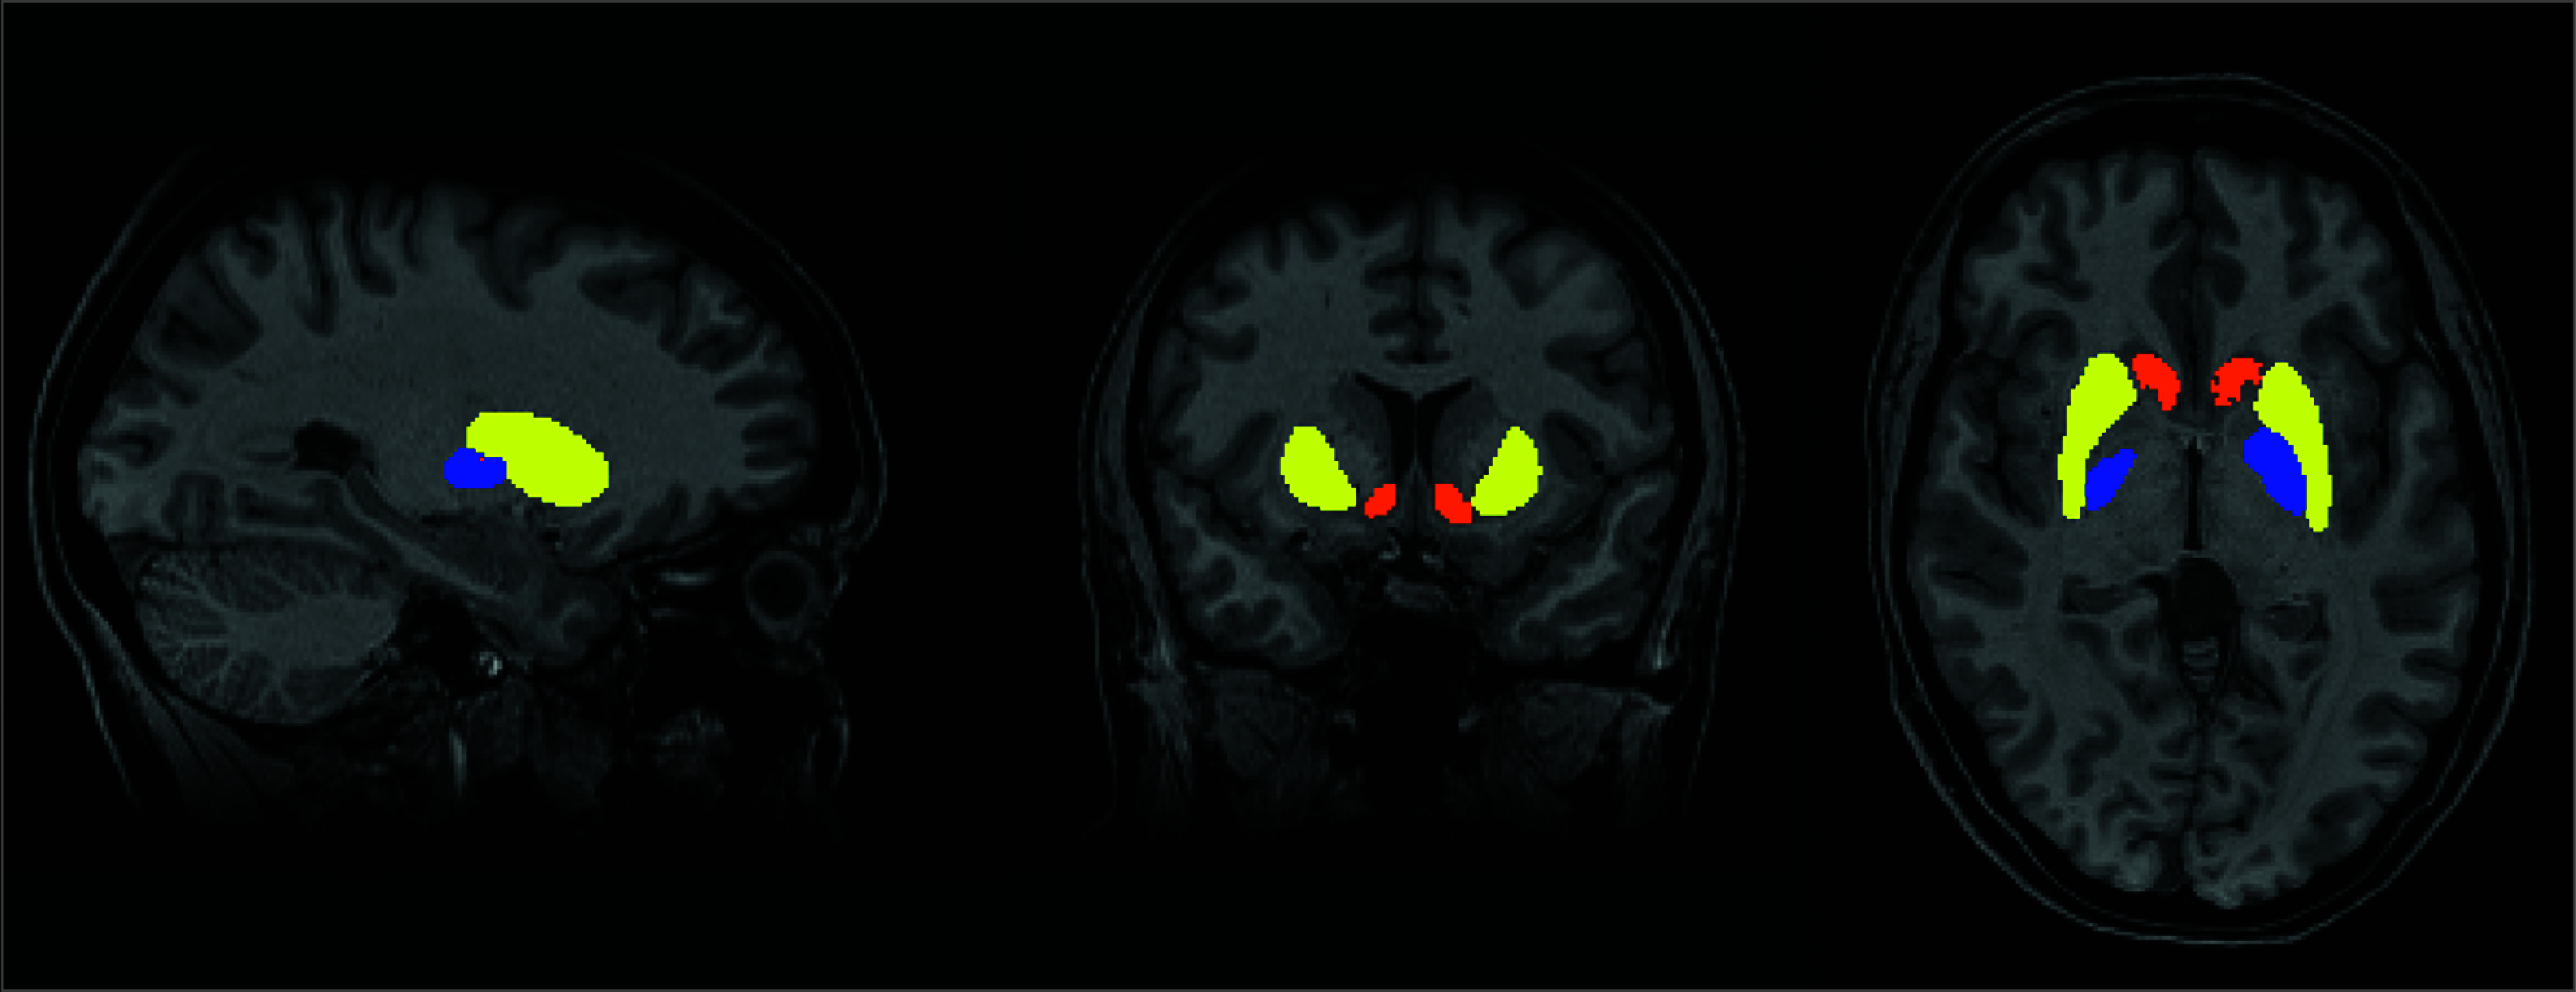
Magnetic Resonance Images of the Segmented Putamen, Accumbens, and Pallidum in a Trial Participant Yellow indicates the putamen; orange, accumbens; and blue, pallidum.
